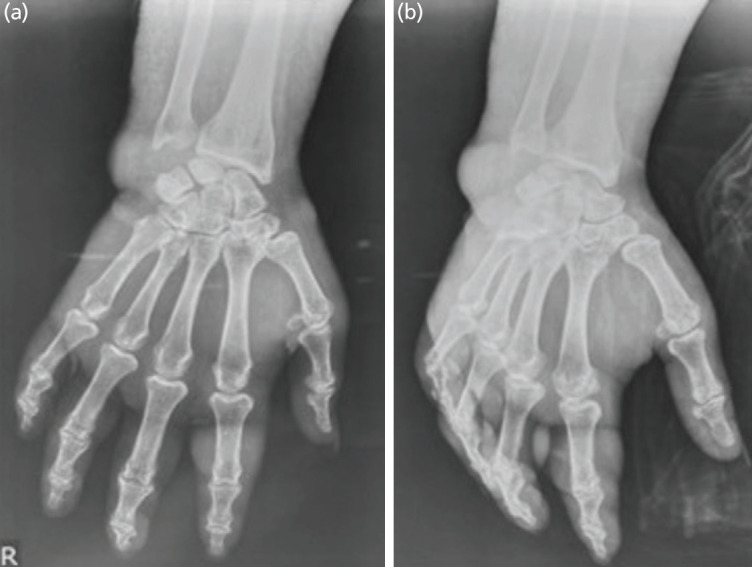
(a and b) Radiograph of the right hand showed soft tissue swelling.
